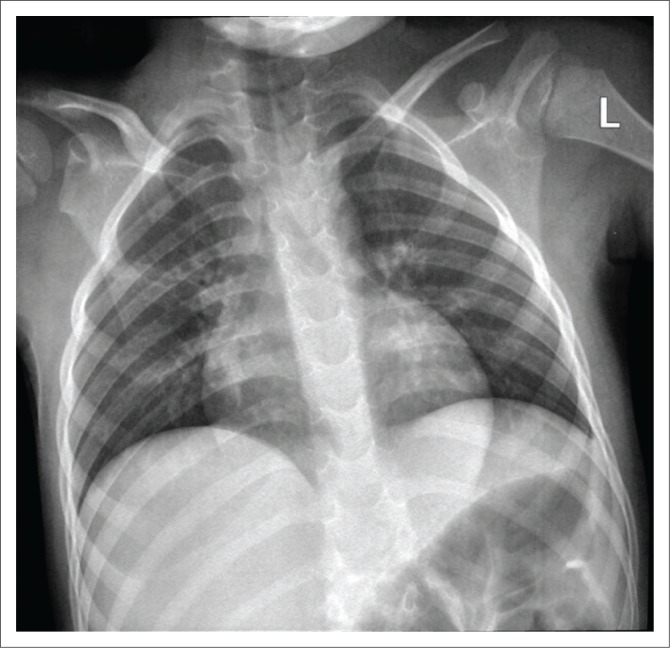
Plain X-ray demonstrating unremarkable features of the chest.
